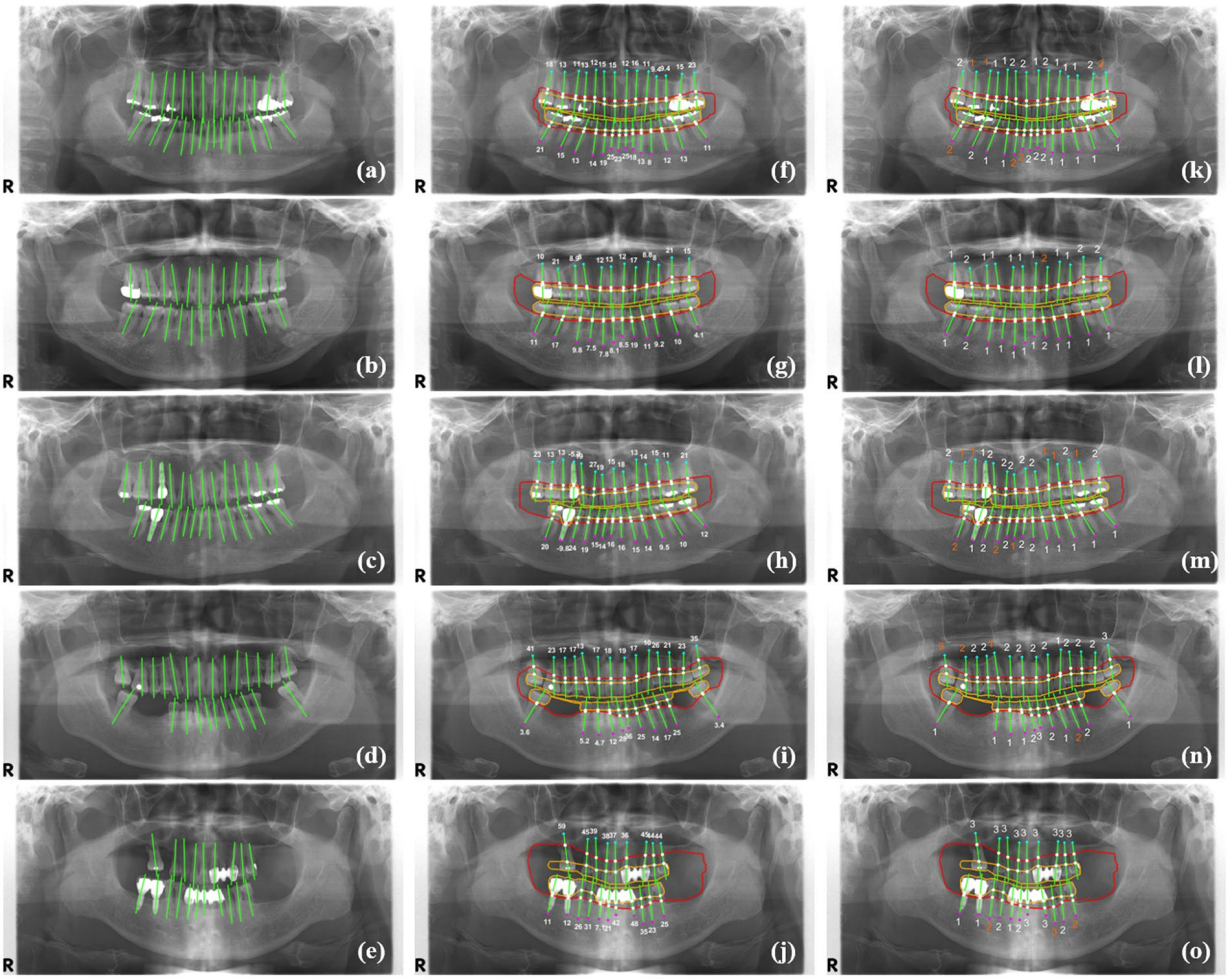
The long-axis orientations of the tooth and the implant (a–e), the intersection points of the tooth (implant) long-axis with the periodontal bone level and the CEJ level (fixture top level), the percentage rate of the radiographic bone loss (f–j), and the stages of the periodontitis for each tooth and implant (k–o) (correctly classified stages in white color, and incorrectly classified stages in orange color).
